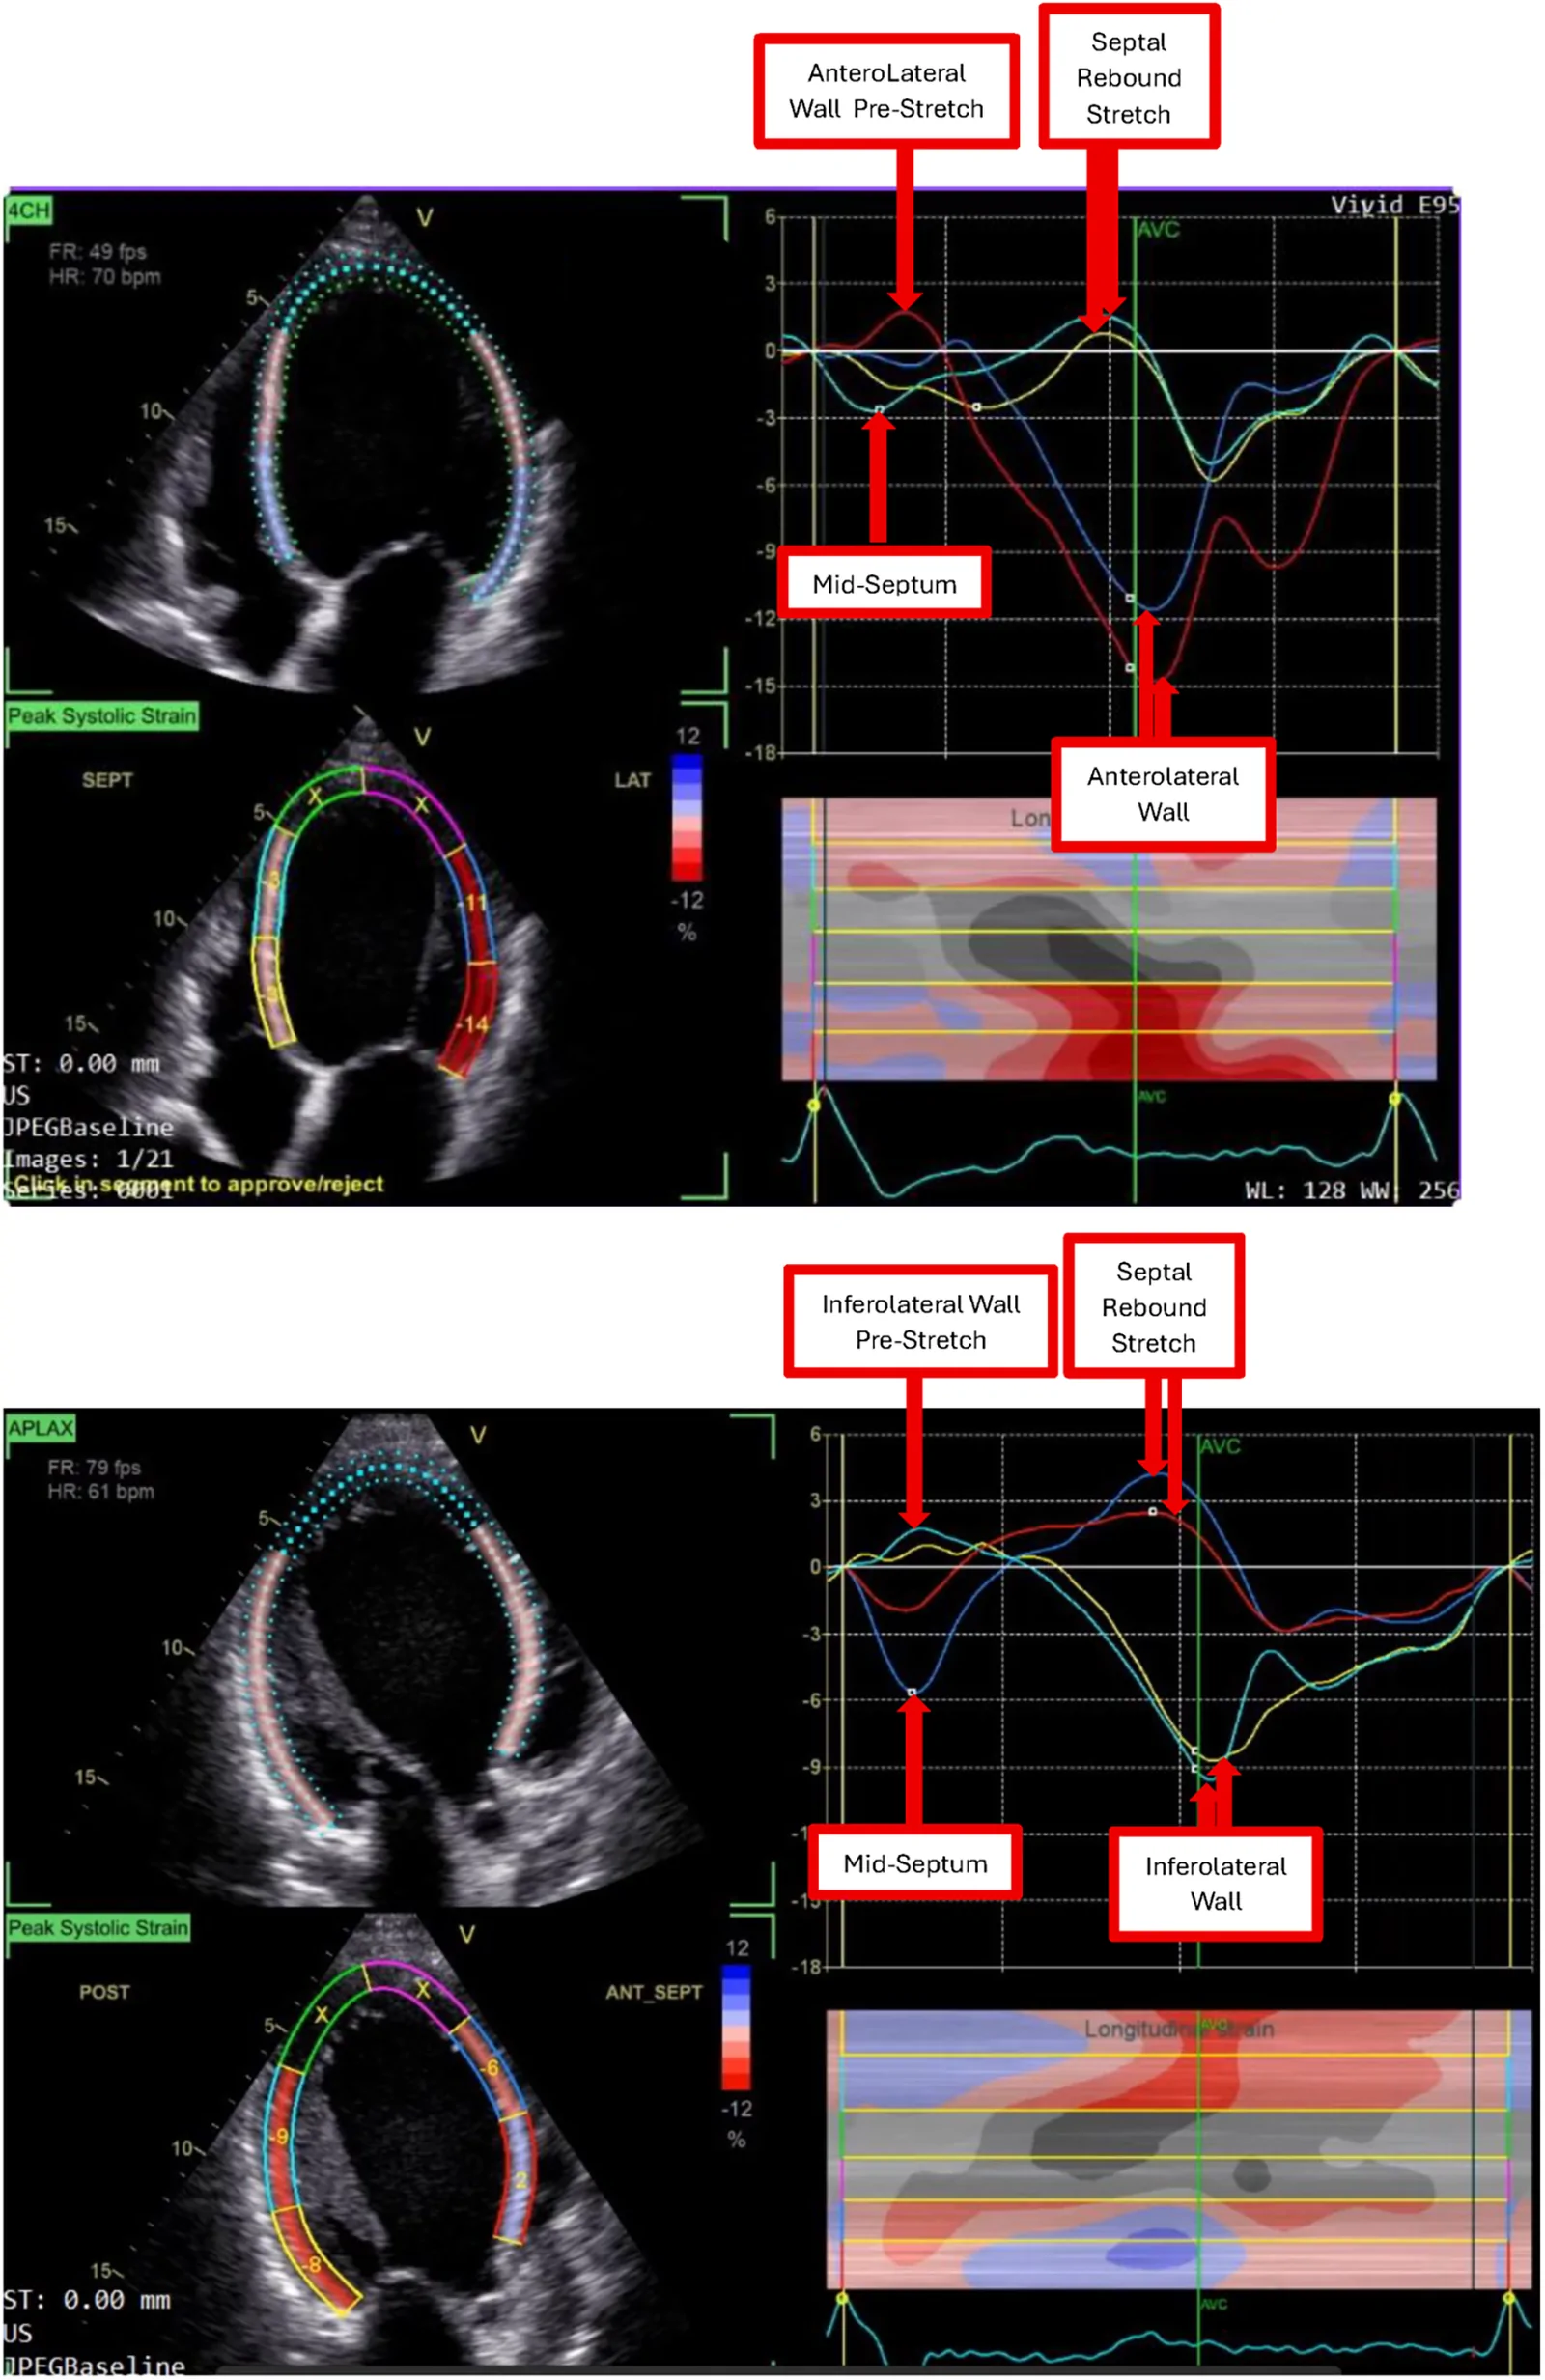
Typical LBBB contraction pattern visualized using 2D-STE in the apical four-chamber (upper panels) and apical long-axis/three-chamber (lower panels) views (for better clarity of the image, the curves for the apical segments were removed). Early peak strain of the septum is followed by continued stretching during the remainder of systole. Similar patterns are observed in 3AC. 2D-STE, two-dimensional speckle-tracking echocardiography; 3AC, apical long-axis (three-chamber) view; 4AC, apical four-chamber view; LBBB, left bundle branch block.
